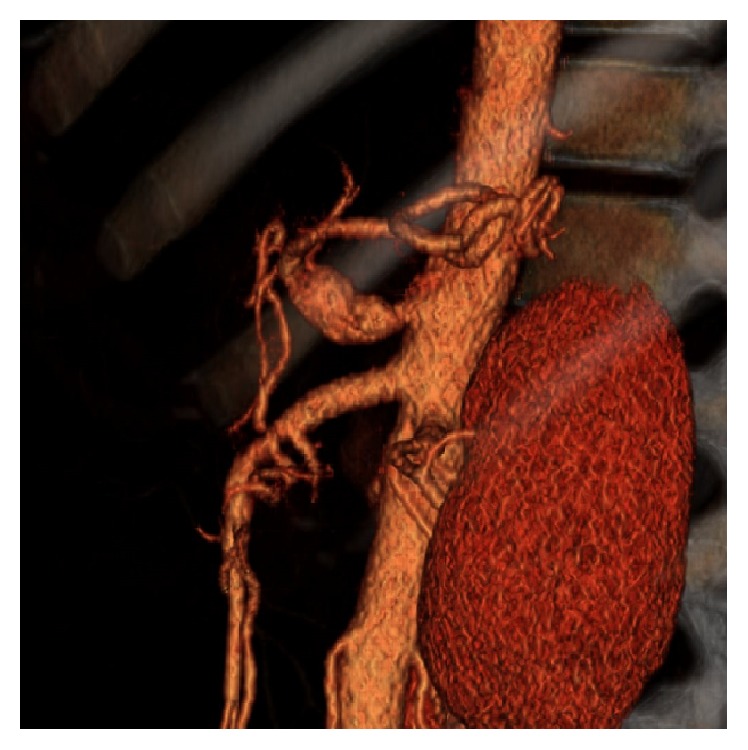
CT angiogram reconstruction of the celiac artery at the time of diagnosis.
